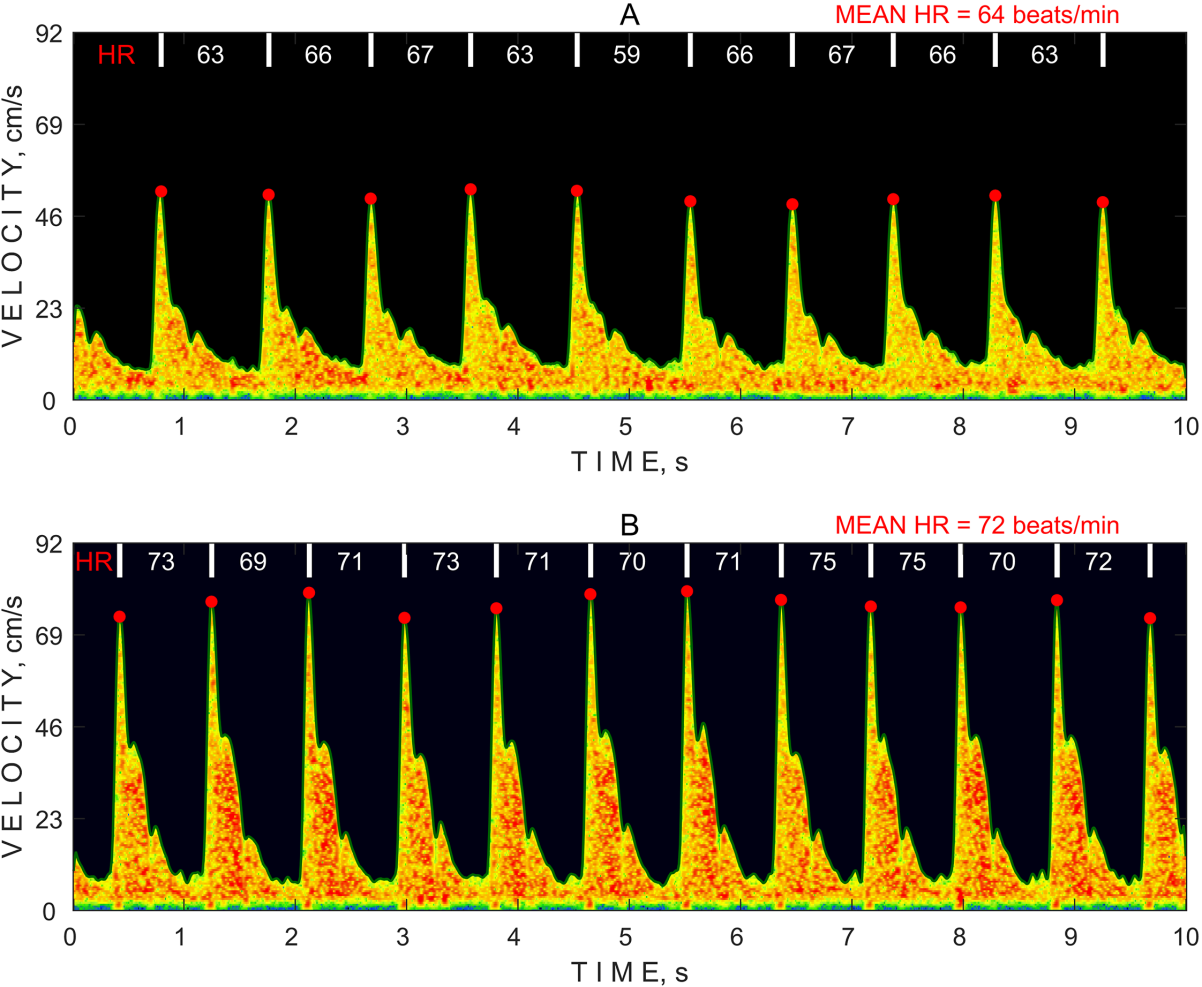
Example spectrograms of pulse waves of blood flow in the ophthalmic artery collected by a transcranial Doppler using different external pressure steps. (A) 0 mmHg; (B) 48 mmHg. HR—heart rate.
